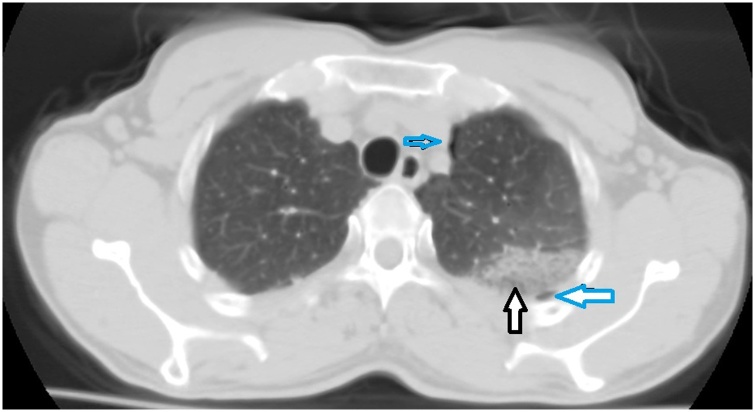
CT axial scan in post traumatic patient with pulmonary contusion (black arrow) and a little pneumothorax (blue arrows), not visible with transthoracic ultrasound, because they are located in areas not accessible to ultrasound (behind the scapula and in the mediastinal pleura).
